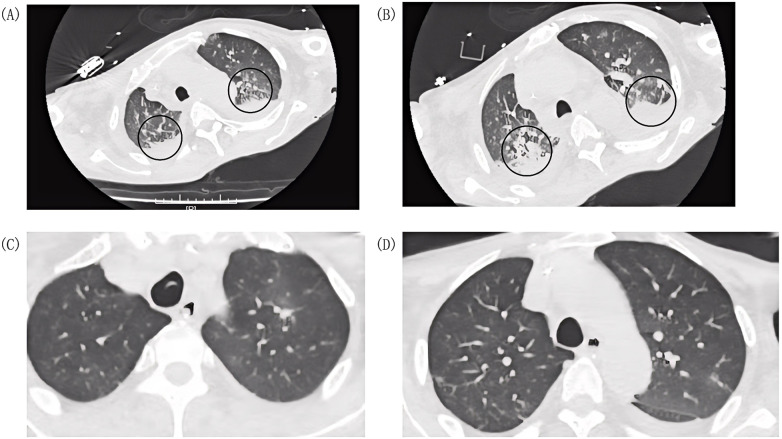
Longitudinal chest CT imaging of the patient. (A,B) Initial unenhanced chest CT scans at admission demonstrate extensive inflammatory involvement across bilateral lung fields. The circled areas highlight characteristic features of invasive pulmonary aspergillosis (IPA), including diffuse ground-glass opacities and dense confluent consolidations, predominantly distributed in the peripheral zones of the lower lobes. (C,D) Follow-up chest CT scans after targeted antimicrobial and antifungal therapy show near-complete resolution of the previous lesions. The lung parenchyma is well-aerated with no residual focal opacities, indicating a substantial radiological improvement and a favorable clinical response.
